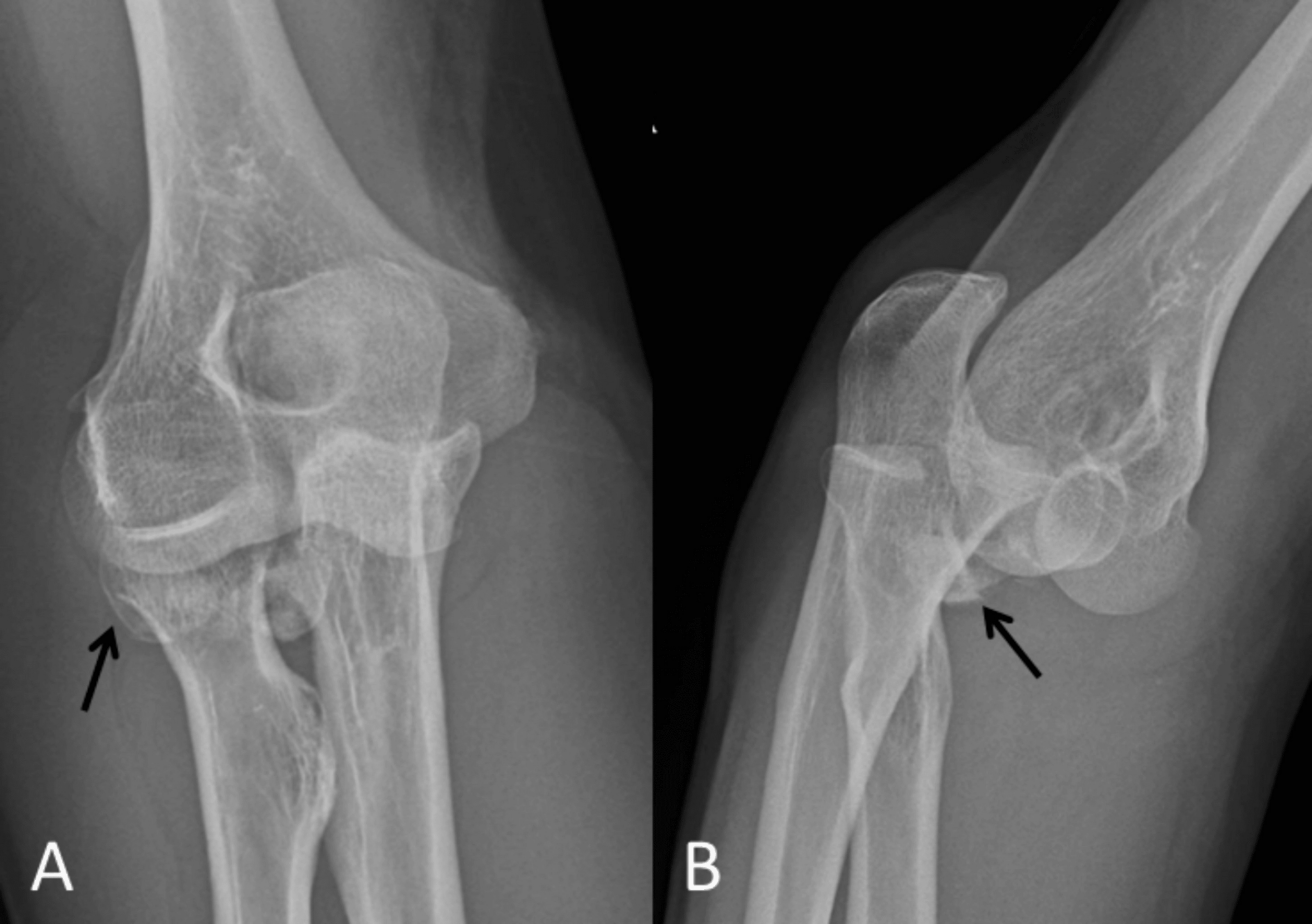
Pre-operative radiographs. A: Antero-posterior view demonstrating a highly comminuted and displaced fracture of the radial head (radial head fragments demonstrated by black arrow). B: Lateral views demonstrating the associated elbow dislocation and one of the displaced radial head fragments (black arrow).
